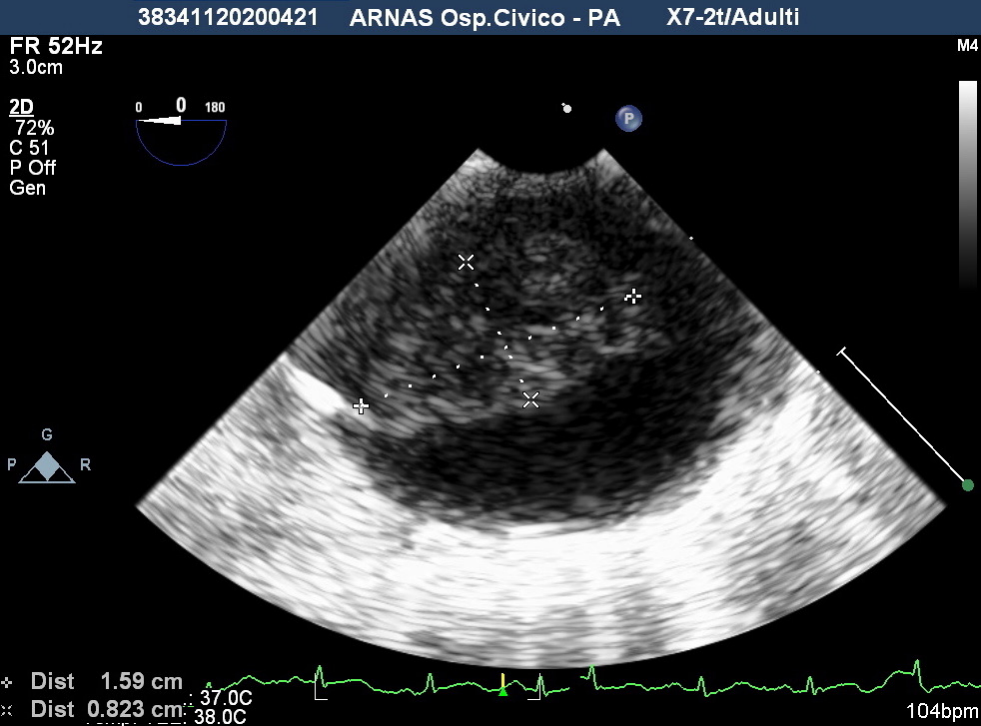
Transesophageal echocardiography showing aortic mural thrombus as pedunculated.
